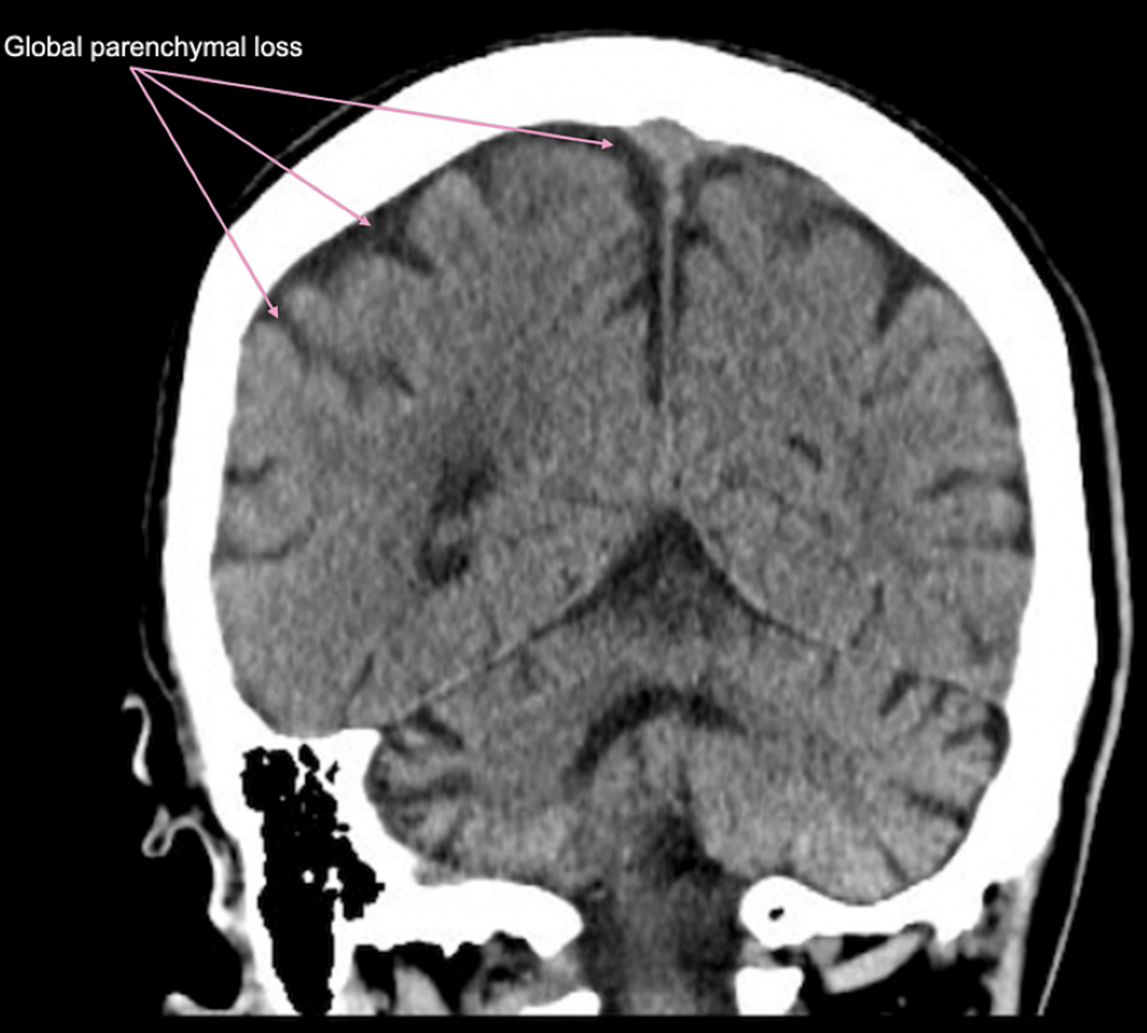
CT head without contrast There was no evidence of acute territorial infarction, parenchymal hemorrhage, or mass. There was mild-moderate global parenchymal volume loss.
